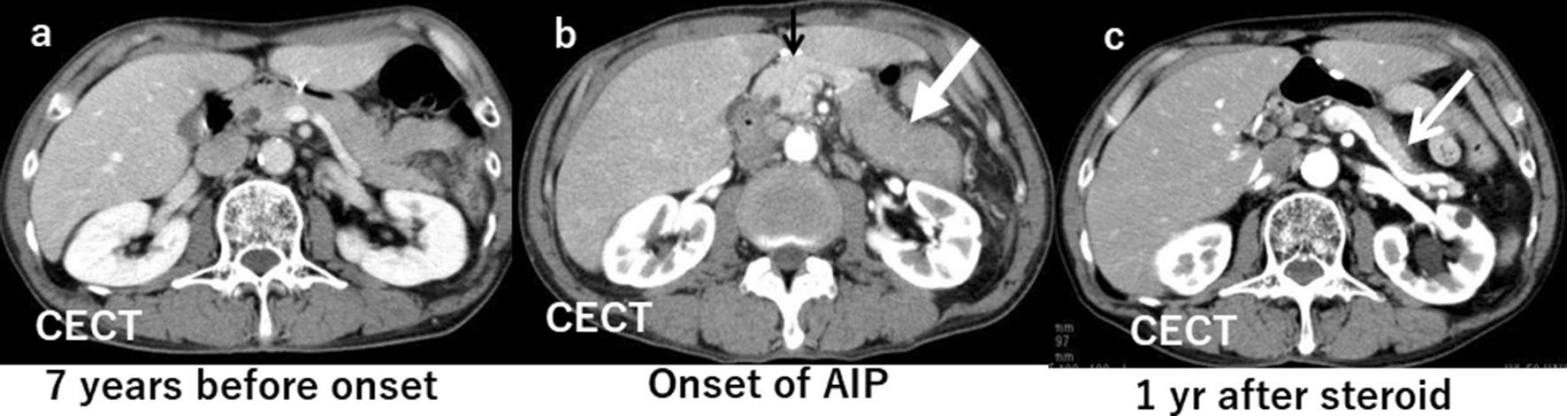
A male patient in his 70 s with autoimmune pancreatitis (AIP). a Seven years before the onset of AIP, there was no pancreatic swelling on contrast-enhanced CT (CECT). b At the onset of AIP, there is distinct sausage-like swelling of the pancreatic body to tail on CECT. The area affected by AIP is a homogeneously less enhanced area (large arrow) as compared to the unaffected area (small arrow). c One year after initiation of prednisolone, the affected lesion returned to a normal size and enhancement on CECT (arrow)
